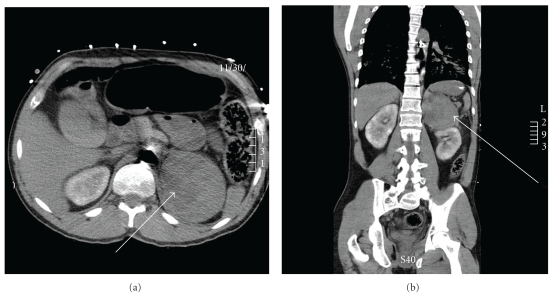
Noncontrast-enhanced abdominal CT scan showing the 9.2 × 7.6 cm soft tissue mass arising from the left adrenal gland (white arrow). (a) Transverse image, (b) Axial image.
